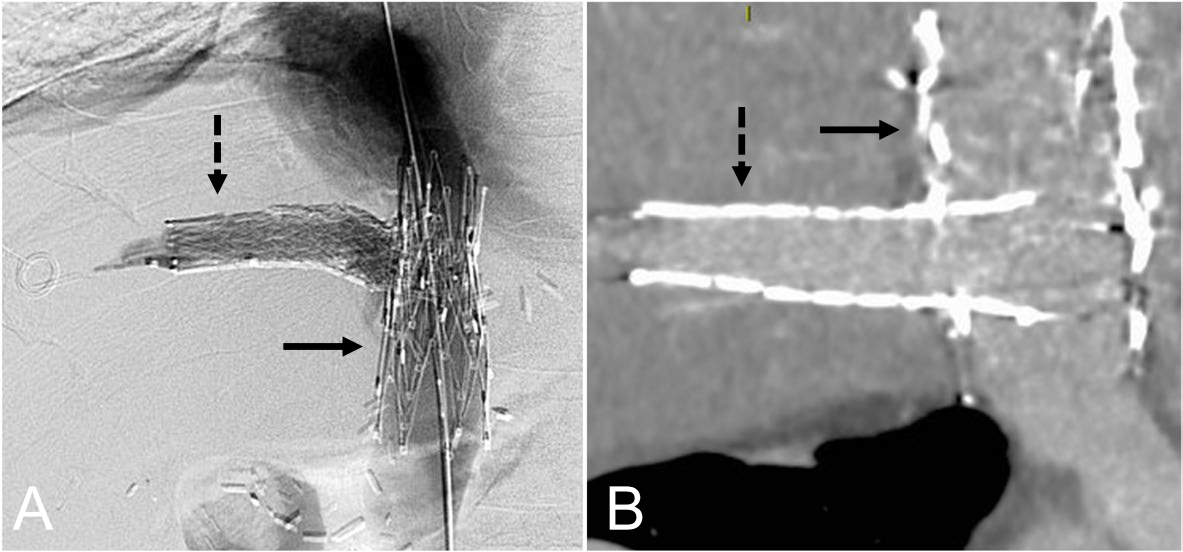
48-year-old male with hepatitis C cirrhosis, status post hepatic transplantation with a piggyback anastomosis presented with ascites, and underwent bifurcated hepatocaval stent reconstruction with two overlapping Z-stents in the IVC [solid arrow] and a Venovo stent in the right hepatic vein [dotted arrow] as seen on diagnostic venography (A) and contrast-enhanced CT (B)
